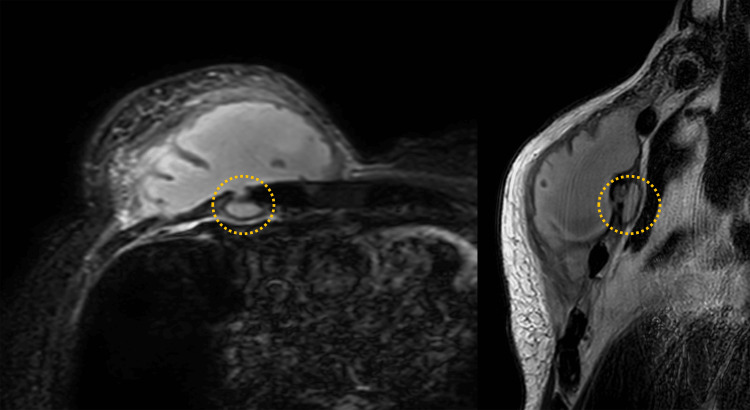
Chest MRI findings. Part of the lesion penetrated the chest wall and formed an abscess (yellow dotted circle) with surrounding pleural thickening.
